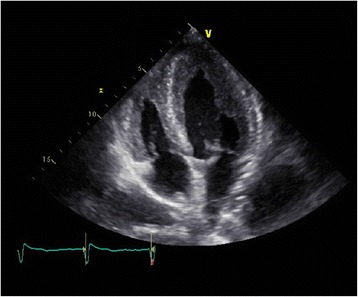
Transthoracic echocardiography, 2 days before initiation of corticosteroid treatment; apical four-chamber view showing biventricular thickening of the myocardium most prominent towards the apex
